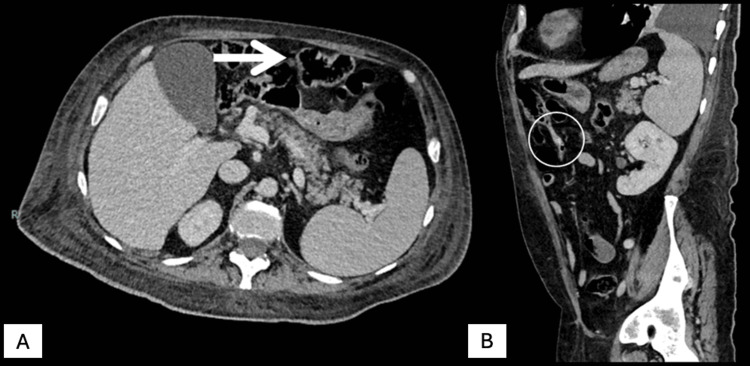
Ectopic cecum Contrast-enhanced CT images in the venous phase. (A) Axial and (B) coronal images demonstrating an ectopic cecum (white arrow) located in the left epigastrium and the appendix (white circle) in the left upper quadrant.
